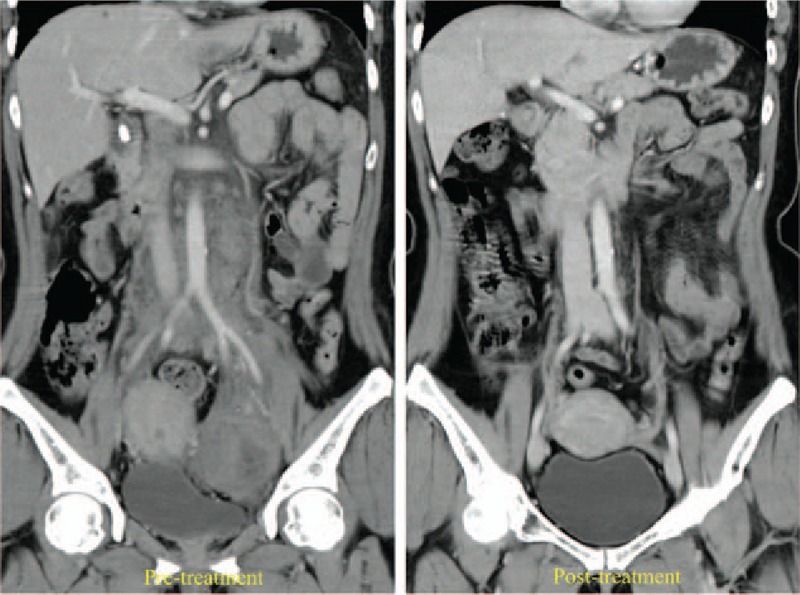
Contrast-enhanced abdominal CT images of the second patient before and after 3 months of everolimus treatment. The pretreatment tumor encased the inferior vena cava and aorta and extended into the pelvis. Complete remission was achieved at 18 months with everolimus 10 mg daily. CT = computed tomography.
